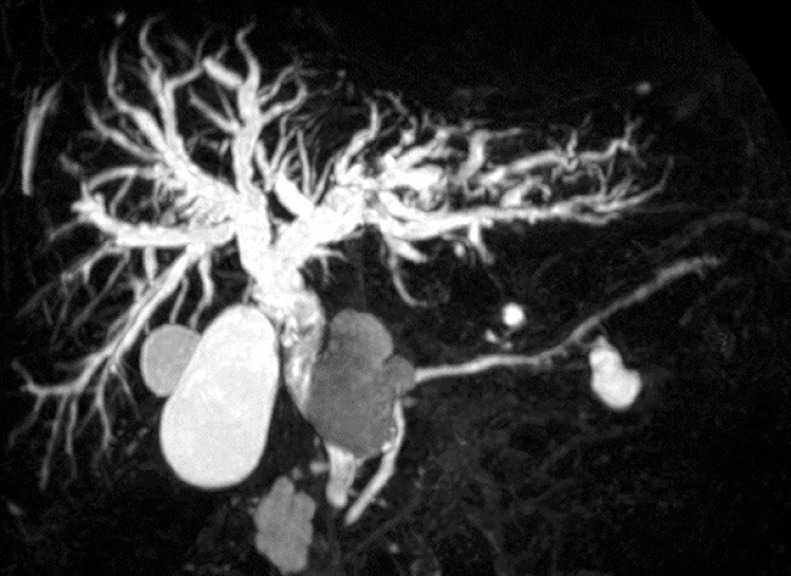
Magnetic resonance imaging showed a large cystic lesion at the pancreatic head and multiple stones at the perihilar bile duct.
